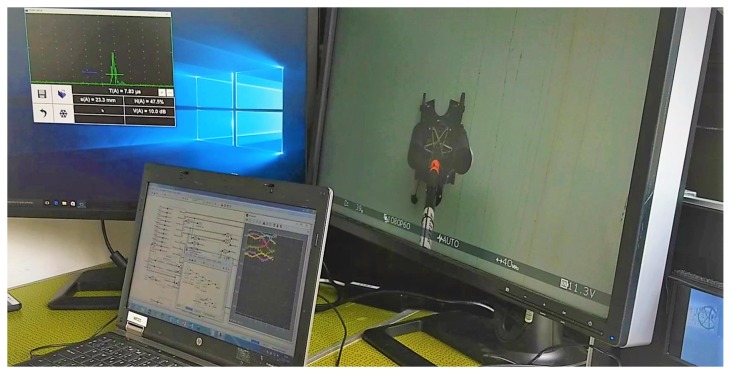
Main three screens visualized by the inspector during operation. On the right screen an onboard camera shows the end-effect and the surface under inspection. The screen on the left is used to manage and visualize the inspection sensors: an ultrasound measurement during this flight experiment is shown. The laptop shows the telemetry and status of AeroX.
